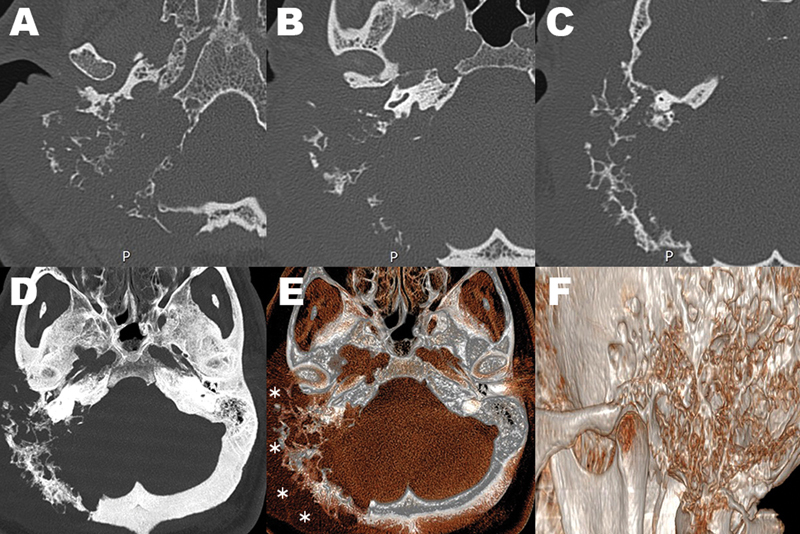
High-resolution computed tomography images of the temporal bones (
